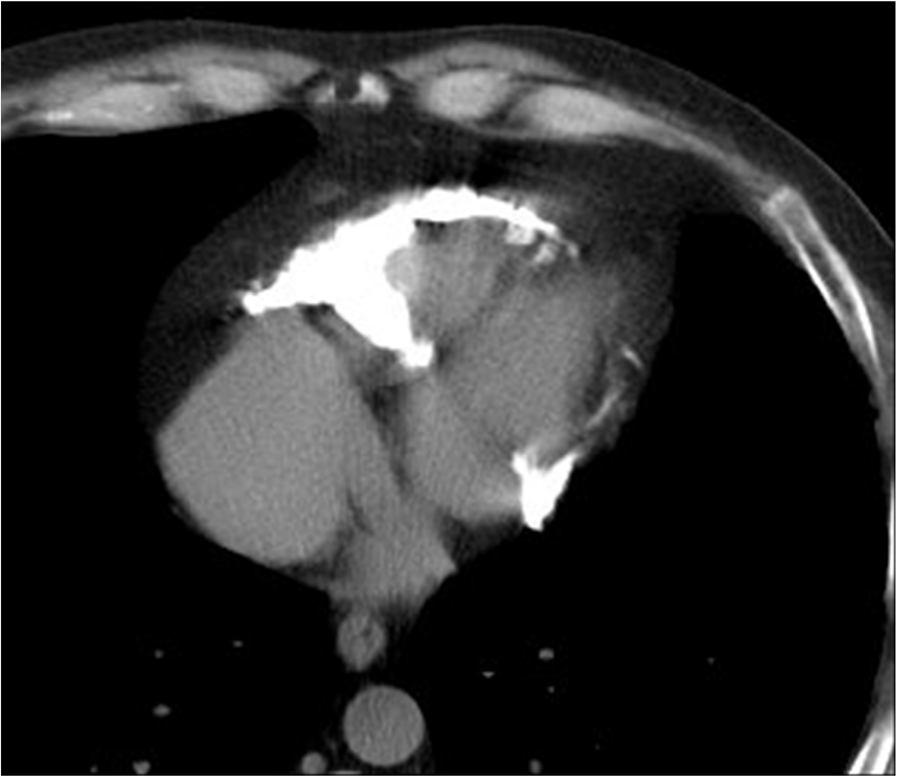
Axial CT image demonstrates chunky pericardial calcification on a non-contrast image, in the AV grooves and bilaterally in a 36-year-old male being investigated for chronic pain
